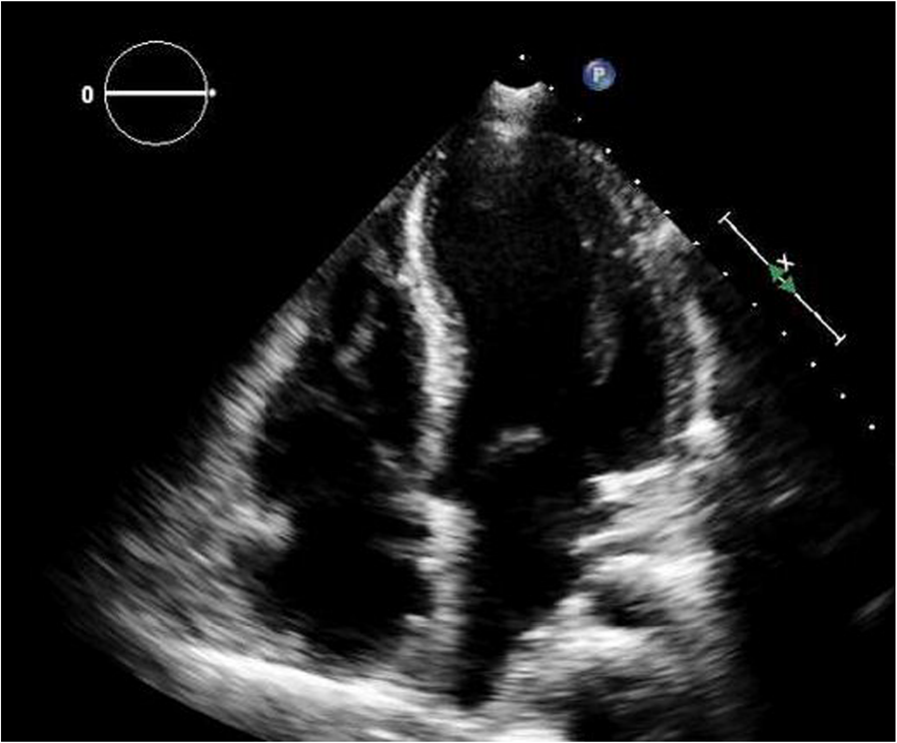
Post-operative transthoracic two-dimensional echocardiography, apical 4-chamber view showing no intra-cardiac masses
